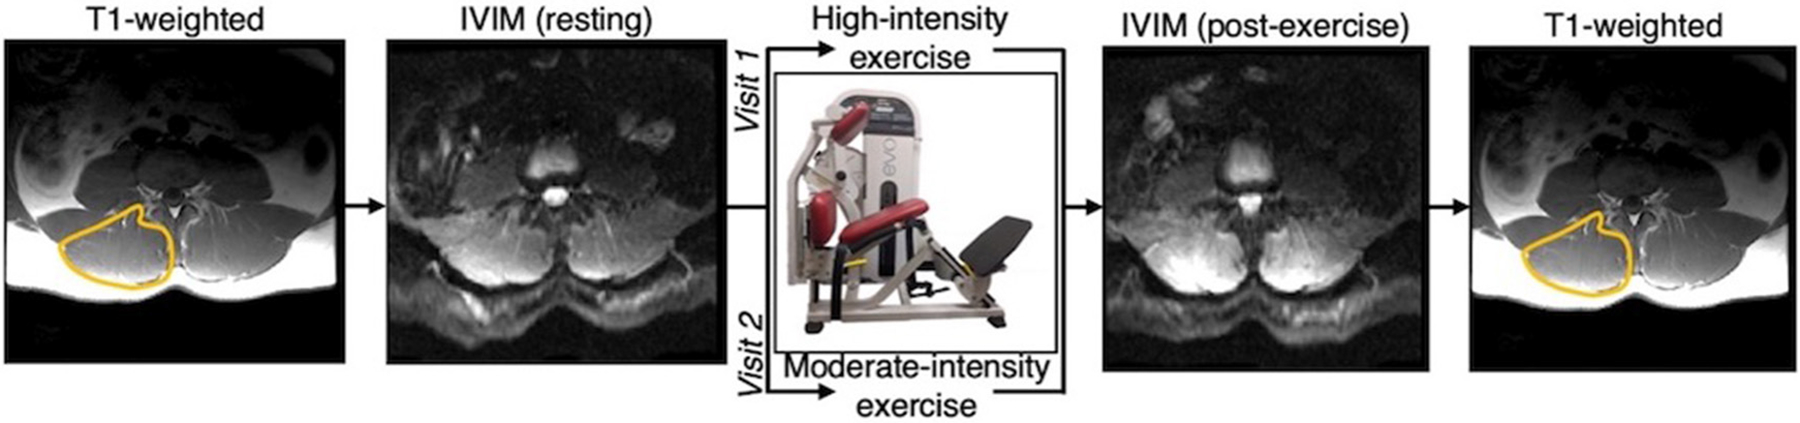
Overview of experimental design. Participants were scanned at rest and following either a moderate or high intensity resisted lumbar extension exercise paradigm. Moderate and high intensity exercise sessions were performed on two separate days. T1-weighted anatomical reference images were acquired before and after the pre- and post-exercise IVIM data, respectively, and used to define the paraspinal muscles bilaterally.
